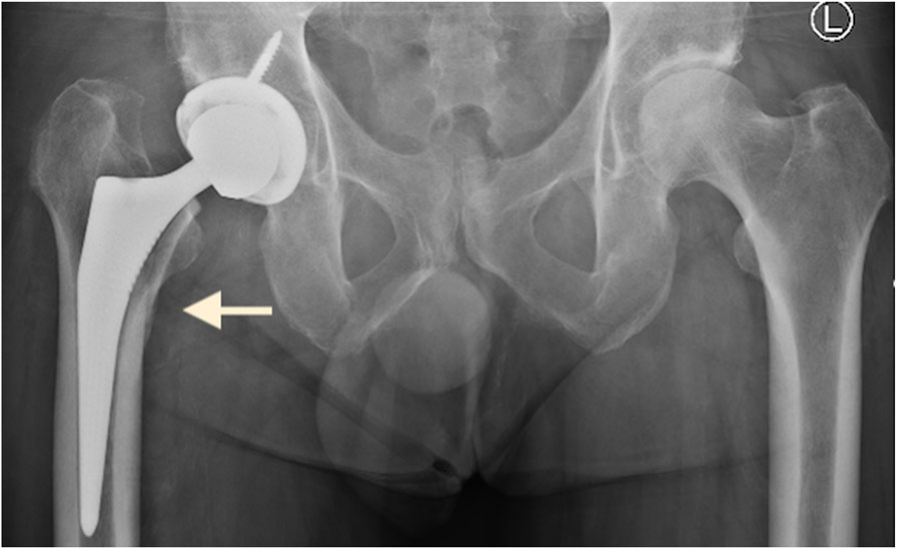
X-ray of femoral fracture with subsidence of stem
